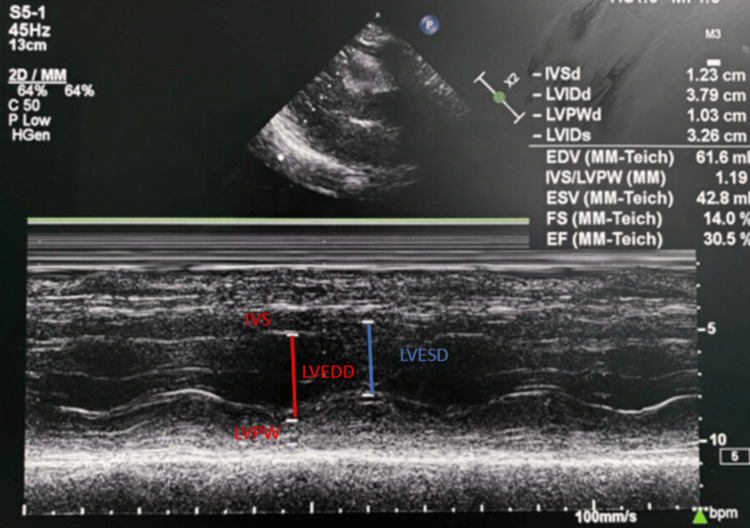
Transthoracic M-mode echocardiography from parasternal long-axis view showing a left ventricular ejection fraction of 30%. IVS = interventricular septum; LVPW = left ventricular posterior wall; LVEDD = left ventricular end-diastolic diameter; LVESD = left ventricular end-systolic diameter
